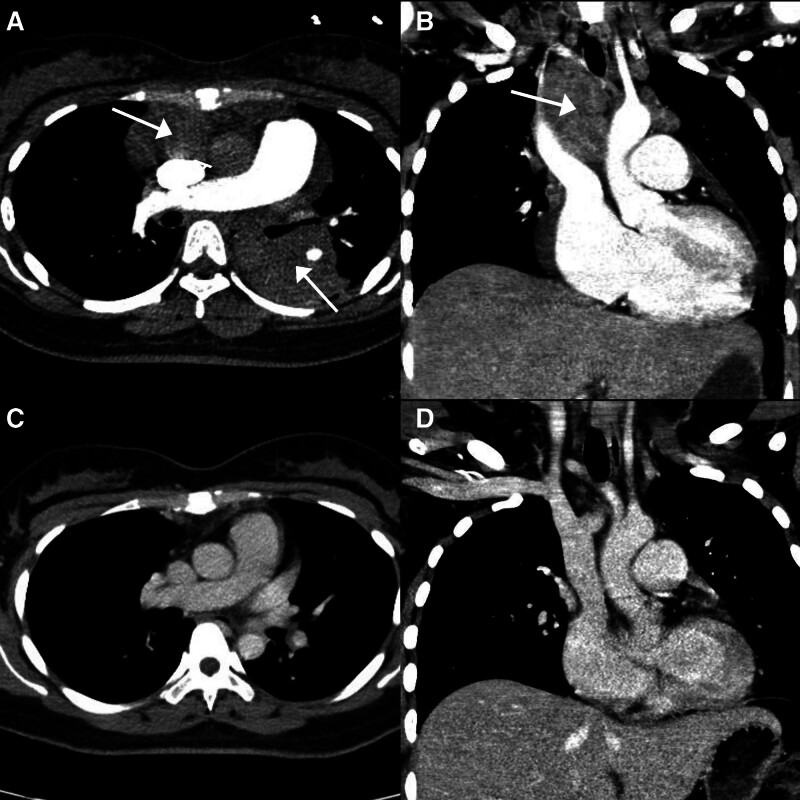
CTPA on January 25, 2024 (A, B) and repeat chest CT on December 19, 2019 (C, D). (A) Transverse image shows left lung mass and multiple mediastinal nodules. (B) Coronal image shows multiple mediastinal nodules. (C, D) CT shows that left lung mass had almost disappeared and multiple enlarged lymph nodes in mediastinum had significantly reduced. CT = computed tomography, CTPA = computed tomography pulmonary angiography.
